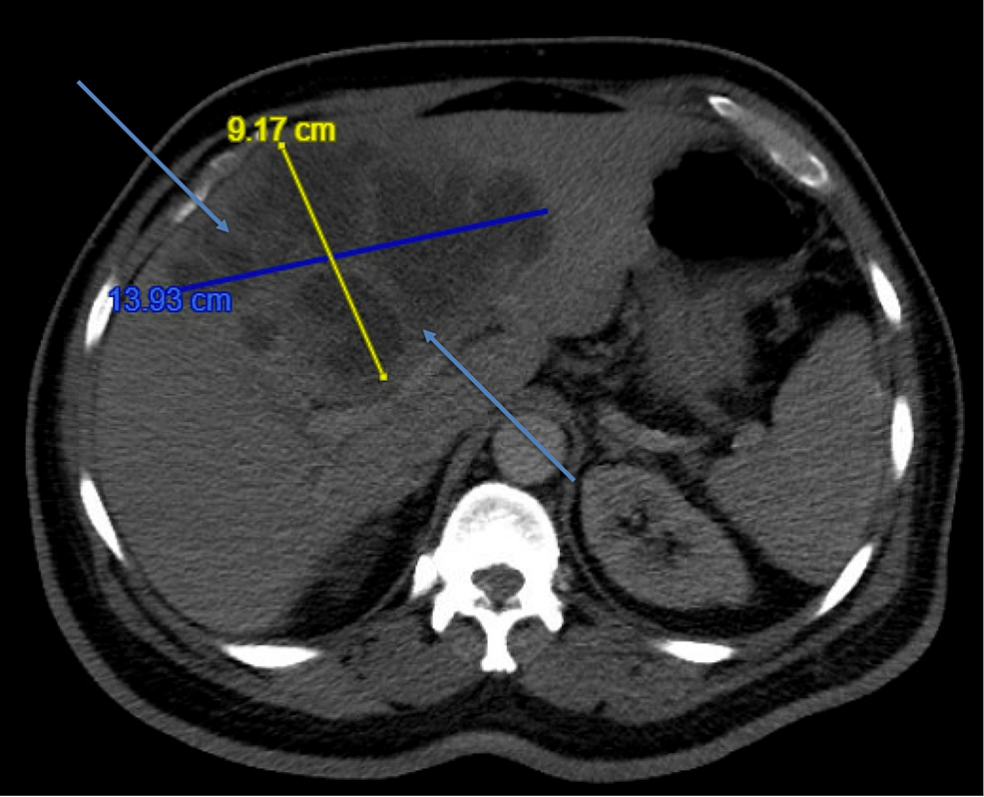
CT scan of the abdomen and pelvis with intravenous (IV) contrast. A 14 cm complex cystic lesion located within the right lobe of the liver, suggesting a high likelihood of being a giant liver abscess.
